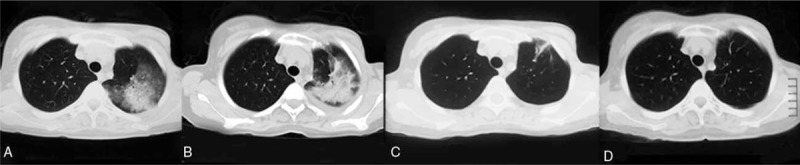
(A) CT showing a left upper lobe consolidation. (B) CT showing the consolidation increased and newly seen small-sized pleural effusion. (C) CT showing the consolidations diminished after steroid treatment for 2 weeks. (D) At 6-month follow-up, CT showing no relapse of pulmonary lesions.
